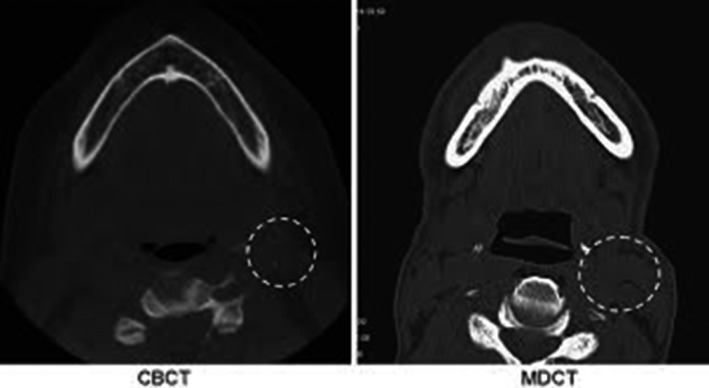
Axial slices through the inferior mandible with CBCT and MDCT shows a loss of soft tissue contrast in CBCT compared to MDCT in the sternocleomastoid region.^38^ Permission was obtained to reproduce these images.
